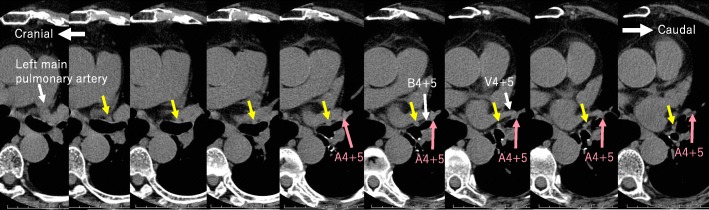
Chest-computed tomography (mediastinal window setting). It shows a pulmonary branch from the left main pulmonary artery to S8 (yellow arrow). It is different from mediastinal A4+5 (pink arrow). It is between the left superior pulmonary vein and the left upper lobe bronchus
